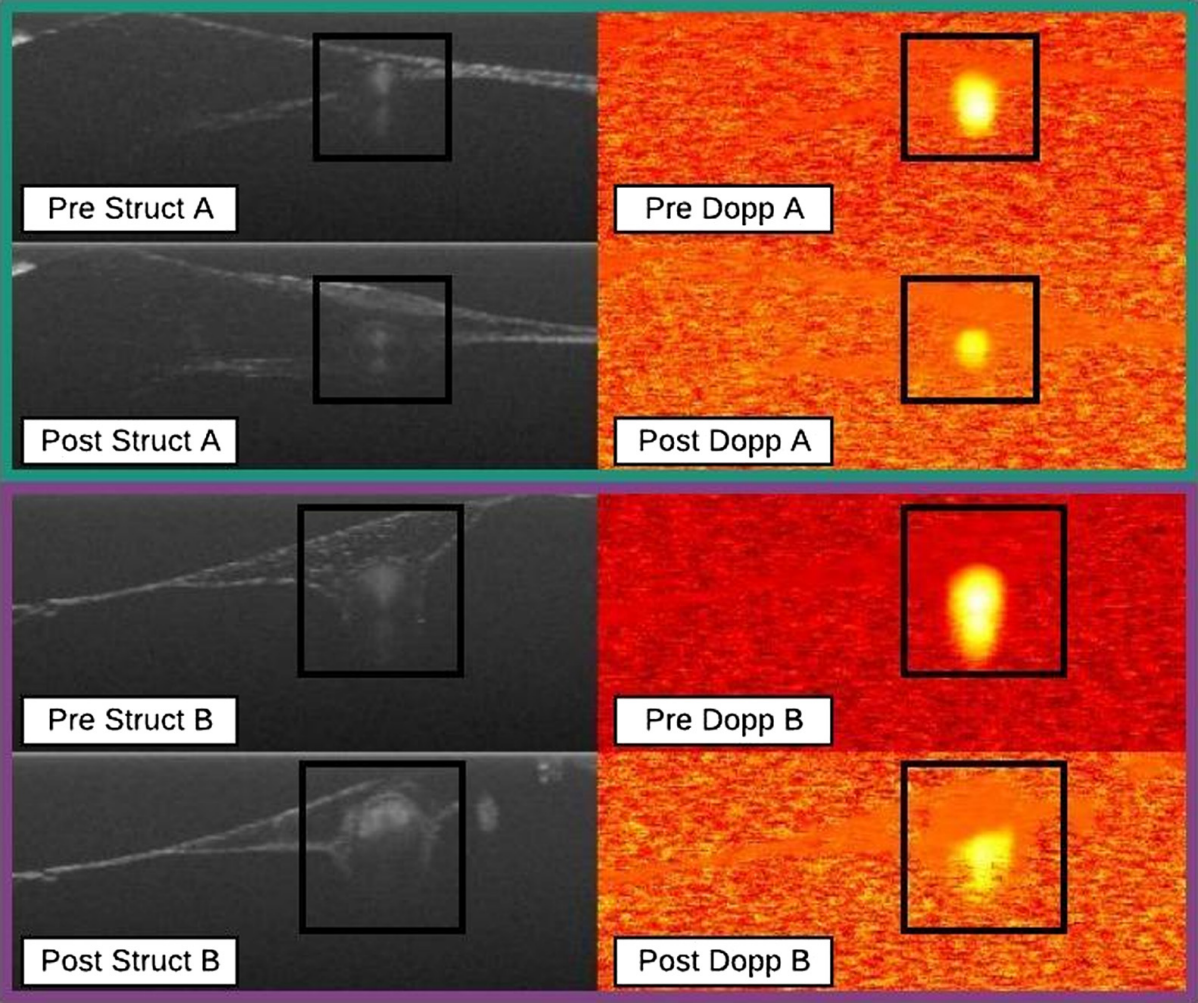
Partial seal data points. Vessel constriction (A, green rectangle) and partial embolism (B, purple rectangle). Black boxes indicate the ROIs. In data point A, the Doppler signal area has clearly been reduced by more than 15% while vessel constriction is apparent from the change in vessel diameter from pre- to poststructural images. In data point B, the Doppler signal area has decreased by more than 15% while from the poststructural image, the homogeneous signal coming from the superficial portion of the vessel lumen accompanied by a lack of a Doppler signal indicates that partial embolism has been achieved.
